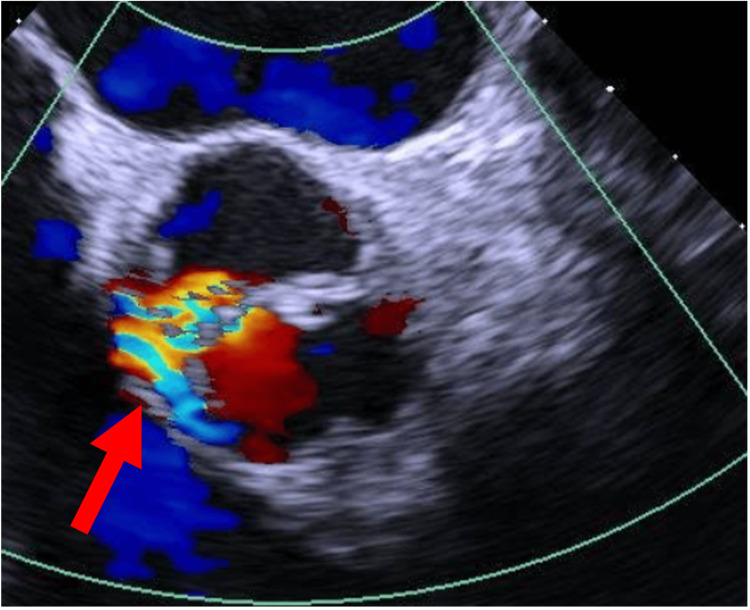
Apparently moderate-grade maximum regurgitation The arrow highlights the aortic regurgitation jet, visualized on color Doppler, indicating high-velocity retrograde blood flow through the aortic valve during diastole.
